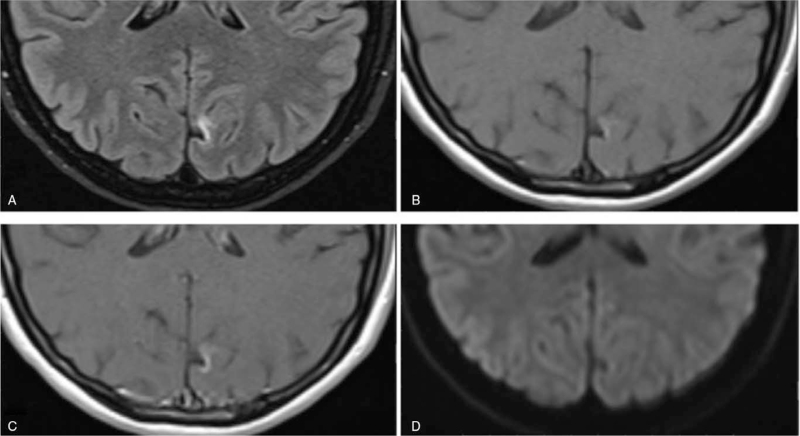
MRI study, axial brain slices of the occipital pole showing ischemic stroke obtained using following sequences: (A) Turbo inversion recovery magnitude (TIRM), (B) T1-weighted turbo spin echo (TSE), (C) T1-weighted TSE after gadolinium contrast administration, and (D) diffusion-weighted imaging (DWI).
